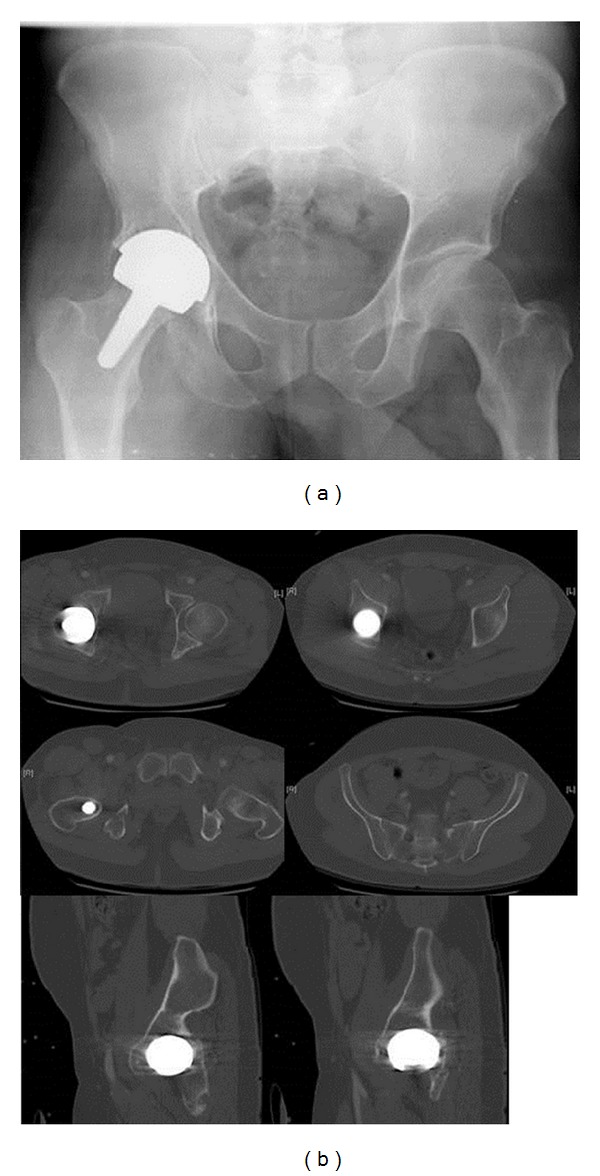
The patient presented with a pelvic ring compression fracture at the right side as well as a periprosthetic posterior column fracture at the left side (emergency X-ray and multislice CT scan).
